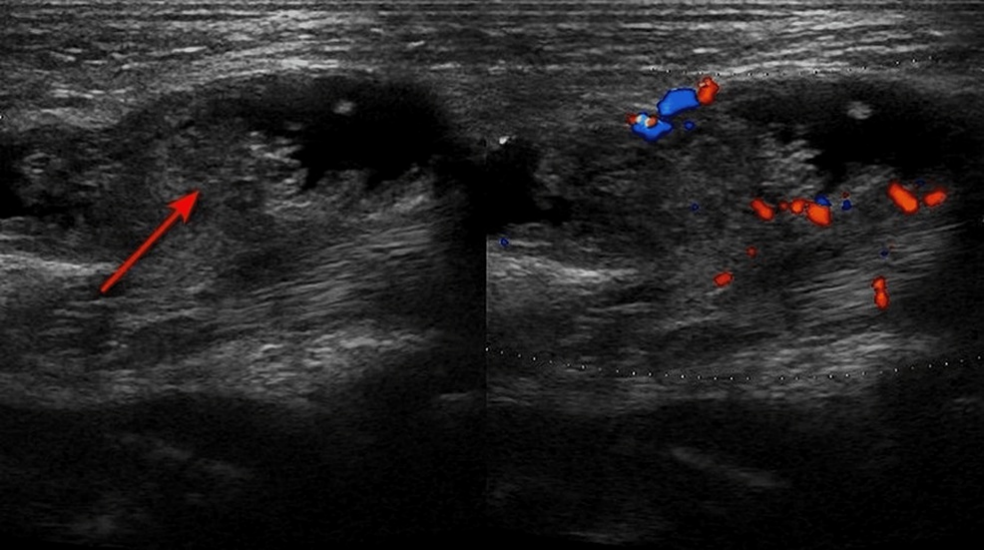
Longitudinal grayscale ultrasound image (left) and color Doppler image (right) of the right knee showing joint effusion with frond-like synovial proliferation in the suprapatellar region (red arrow) showing minimal to no vascularity in the synovial proliferation.
